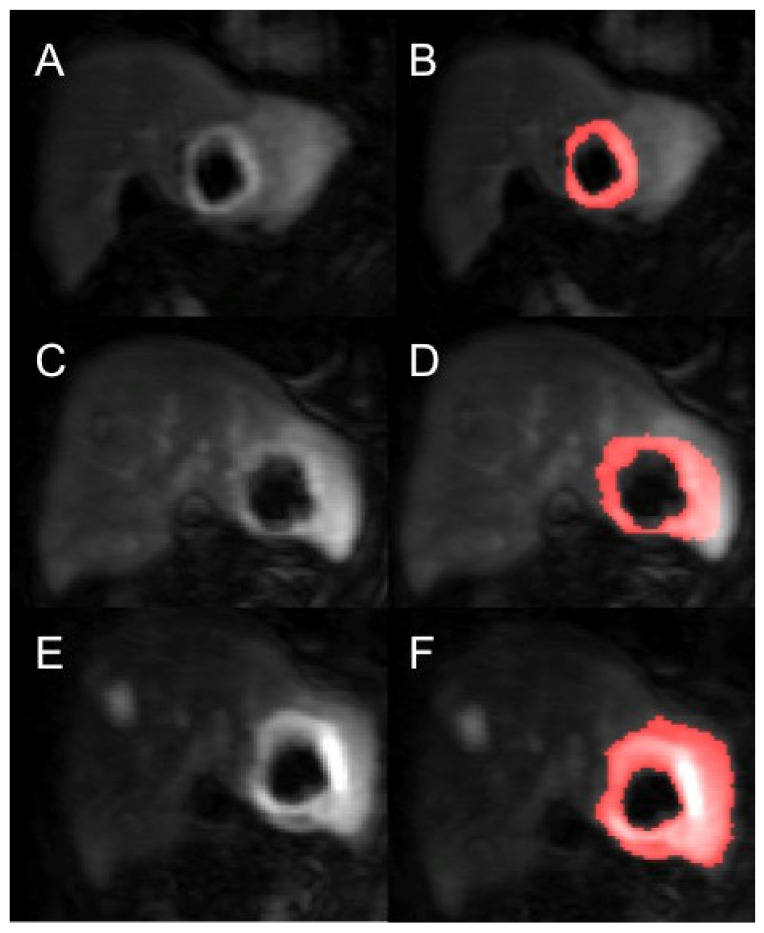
Example of a segmented renal metastasis on DCE-MRI within the left lobe of liver (before and after segmentation with thresholding technique of tumour ROI—the red highlighted area is the tumour ROI segmented on the PMI software) at baseline (A,B), 4-weeks (C,D) and 10-weeks (E,F). This is a case of disease progression at 6 months.
